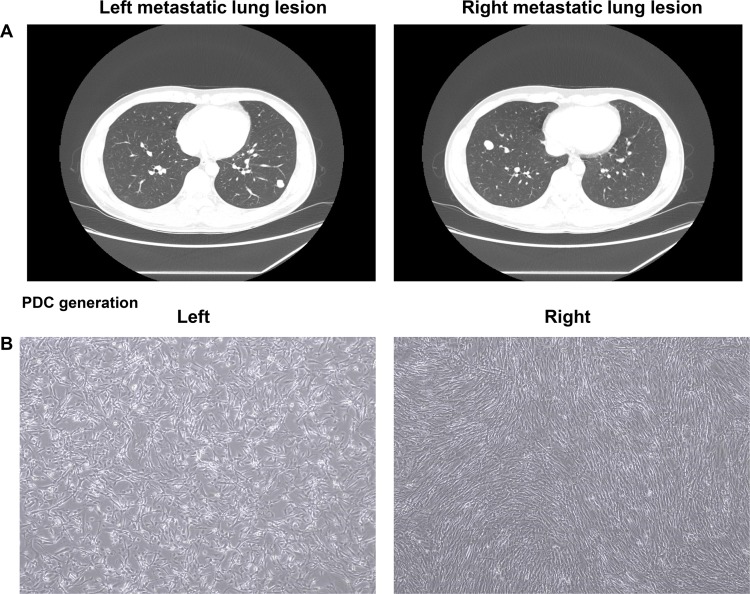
CT scan of the chest in giant cell tumor patient with left and right metastatic lung lesions (A). PDCs originated from left and right metastatic lung lesions, respectively (B).
